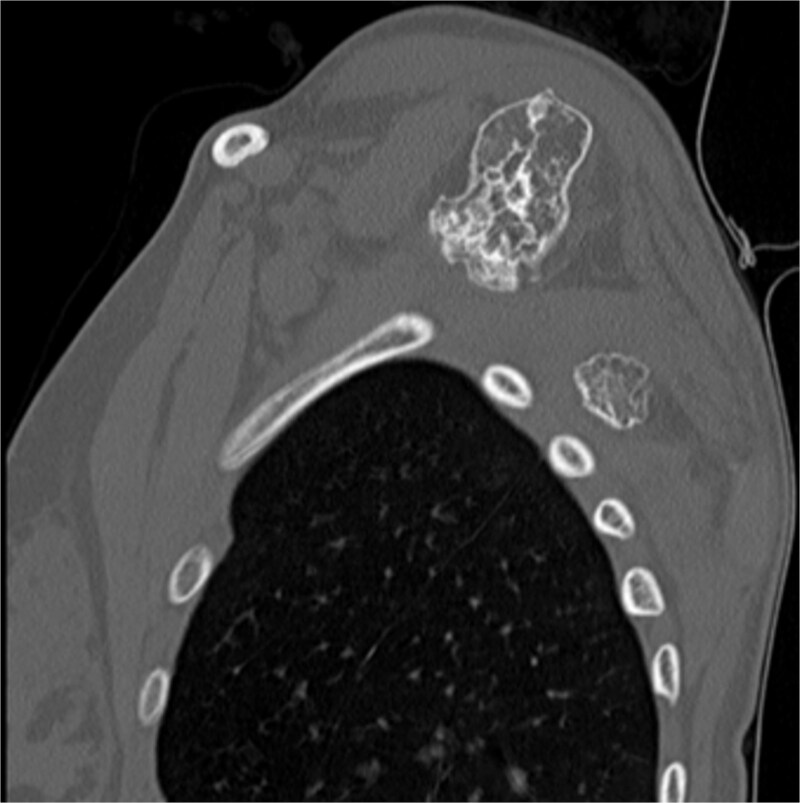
CT of the 14-year-old patient showing both lesions in sagittal plane.
